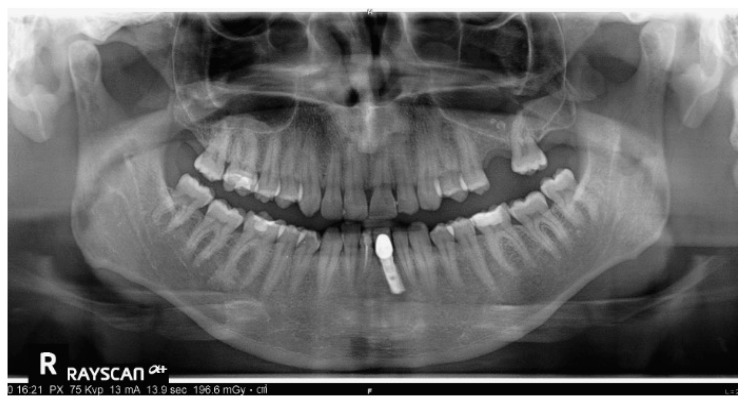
Absence of element 26, implant-prosthesis of element 31, and condylar asymmetry. It was indicated to investigate the diagnostic with an X-ray of elements 36 and 46.
